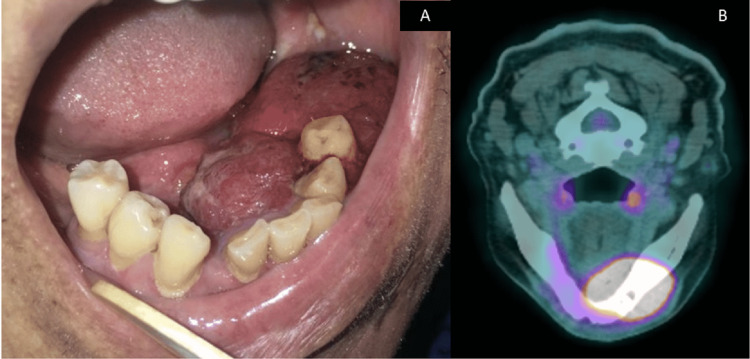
(A) Intraoral photograph shows a large growth involving the left side floor of the mouth extending from the midline to the edentulous molar region. (B) Photograph shows PET-CT image (axial cut) of the primary tumor in the left side body of the mandible PET-CT: positron emission tomography-computed tomography
